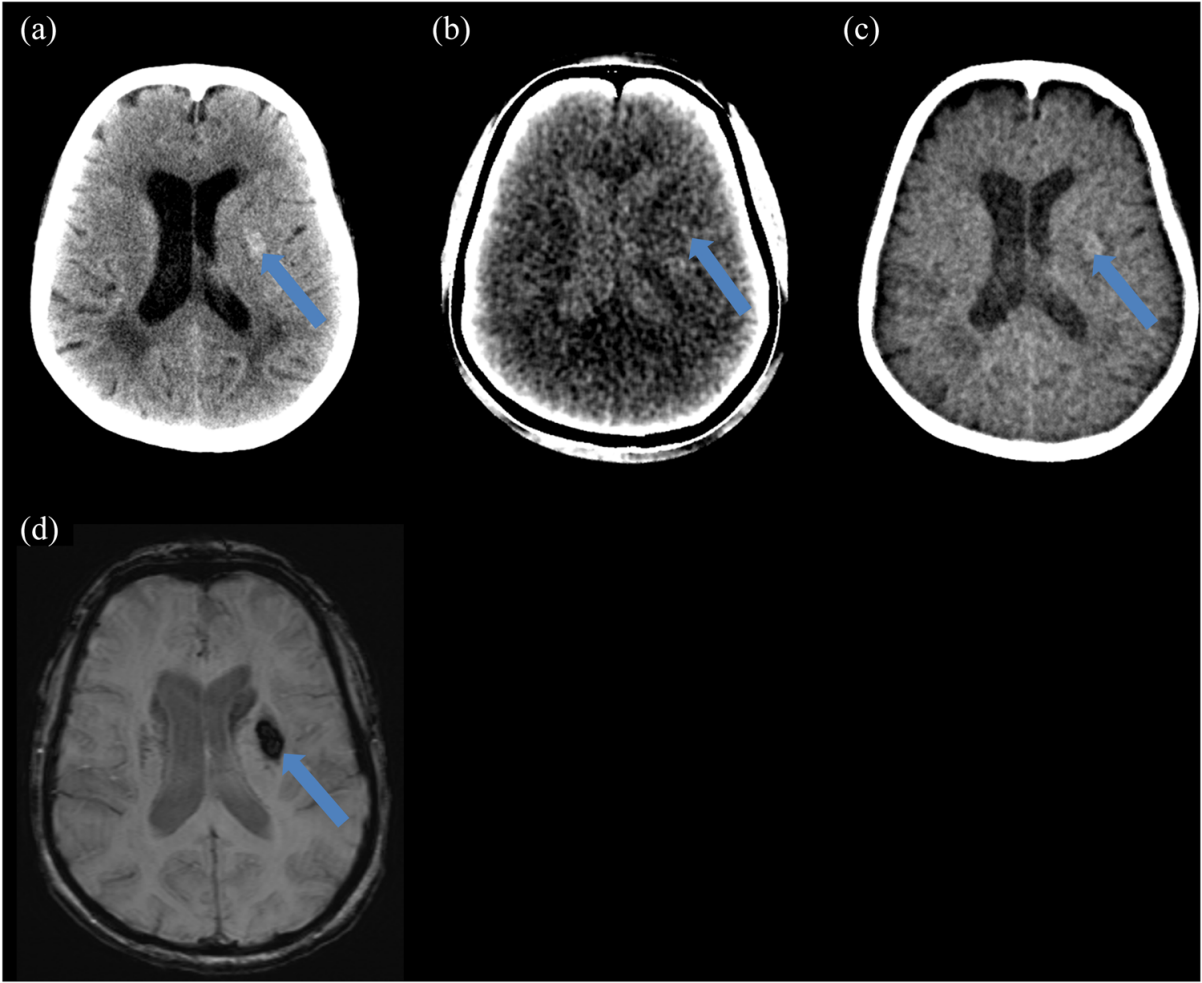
Example of a class 1 hemorrhage agreement. Female 71 years old with a confluent petechial hemorrhage (denoted by arrows) after mechanical thrombectomy. Magnetic resonance imaging (MRI) was obtained approximately 13 h and 27 min after dual energy computed tomography (DECT). (a) 66 keV image shows both contrast extravasation and hemorrhage (arrow), (b) iodine map is negative for contrast extravasation due to a lack of hyper density by the area indicated by the arrow, (c) virtual non‐contrast image showing the hemorrhage as a faint hyper density (arrow), (d) MRI susceptibility weighted imaging (SWI) showing hemorrhage as a hypodense structure (arrow).
